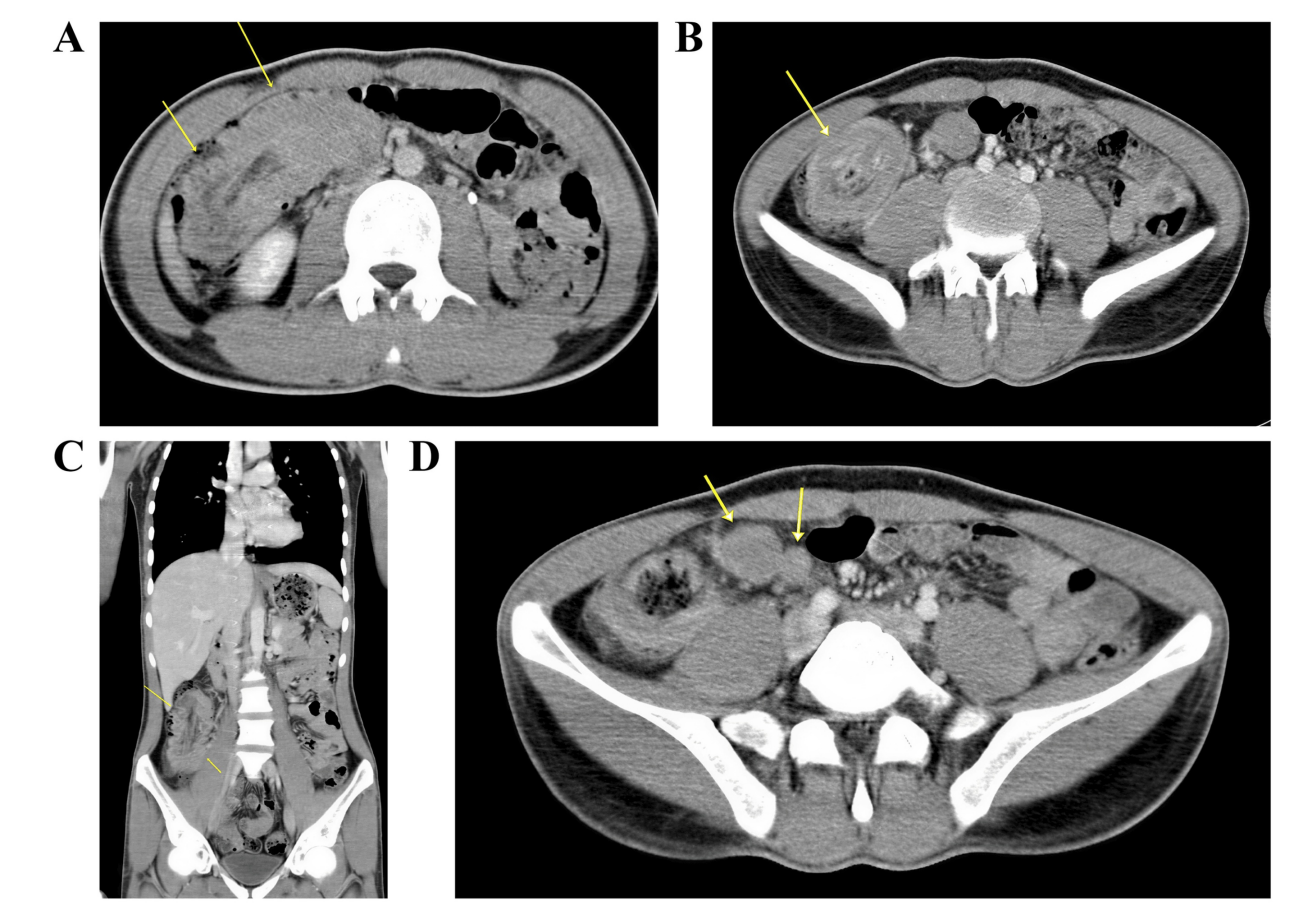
Contrast-enhanced CT scan (A) Axial image showing aneurysmal dilatation of the cecum with diffuse, marked mural thickening (yellow arrows). (B) Axial view demonstrating a mass-like concentric mural thickening involving the ileocecal region (yellow arrow). (C) Coronal reconstruction showing segmental mural thickening of the terminal ileum and cecum (yellow arrow). (D) Axial image displaying enlarged locoregional mesenteric lymph nodes (yellow arrows).
